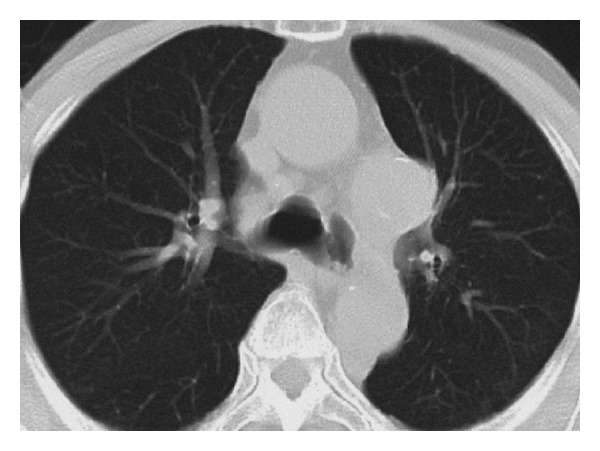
Transverse CT of the chest showing an aortic saccular aneurysm, protruding downward, in the aortopulmonary window.
